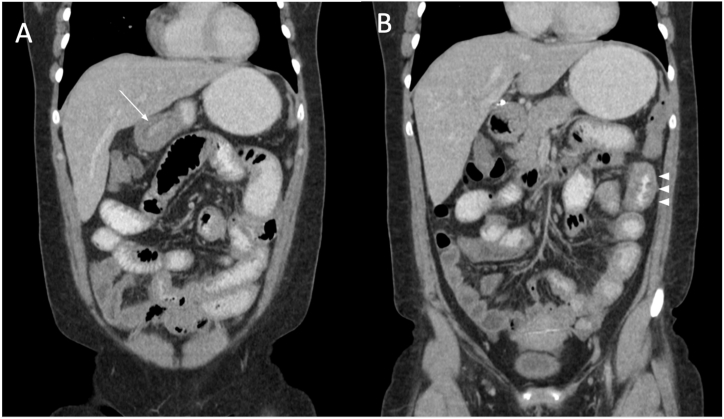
“Abdominal CT scan, coronal plane”. The main findings in the abdominal CT were segmental, concentric and multifocal thickening of small bowel, A. with greater involvement of the pylorus (white arrow) and B., proximal jejunum (arrowheads), causing a slight obstructive effect. A small amount of ascitic fluid was found in the pelvis, not susceptible for drainage.
